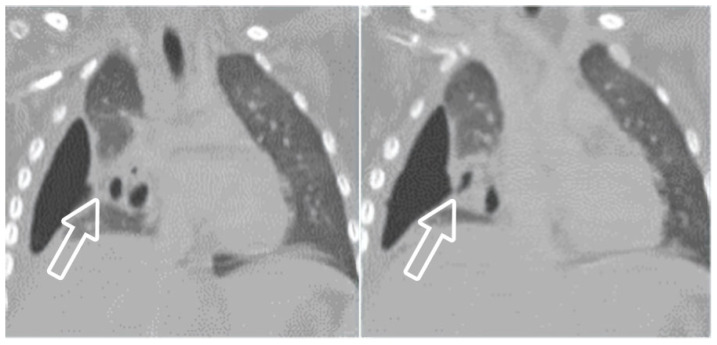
A 4-year-old girl with complicated pneumonia and a surgically confirmed broncho-pleural fistula. CT scan and coronal reconstruction show right loculated pneumothorax and collapsed lung parenchyma, and suggest the fistula to be an indentation (arrow) at the visceral pleura adjacent to a pneumatocele.
